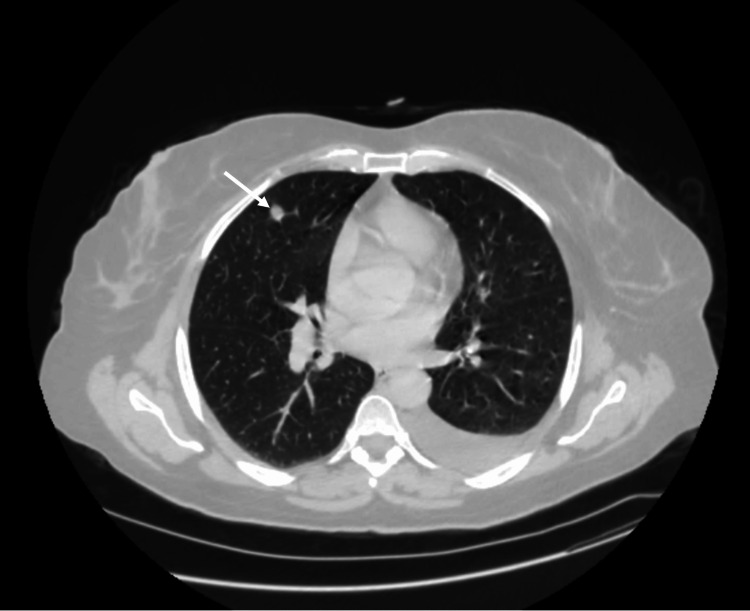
CT scan image showing a pulmonary metastasis
